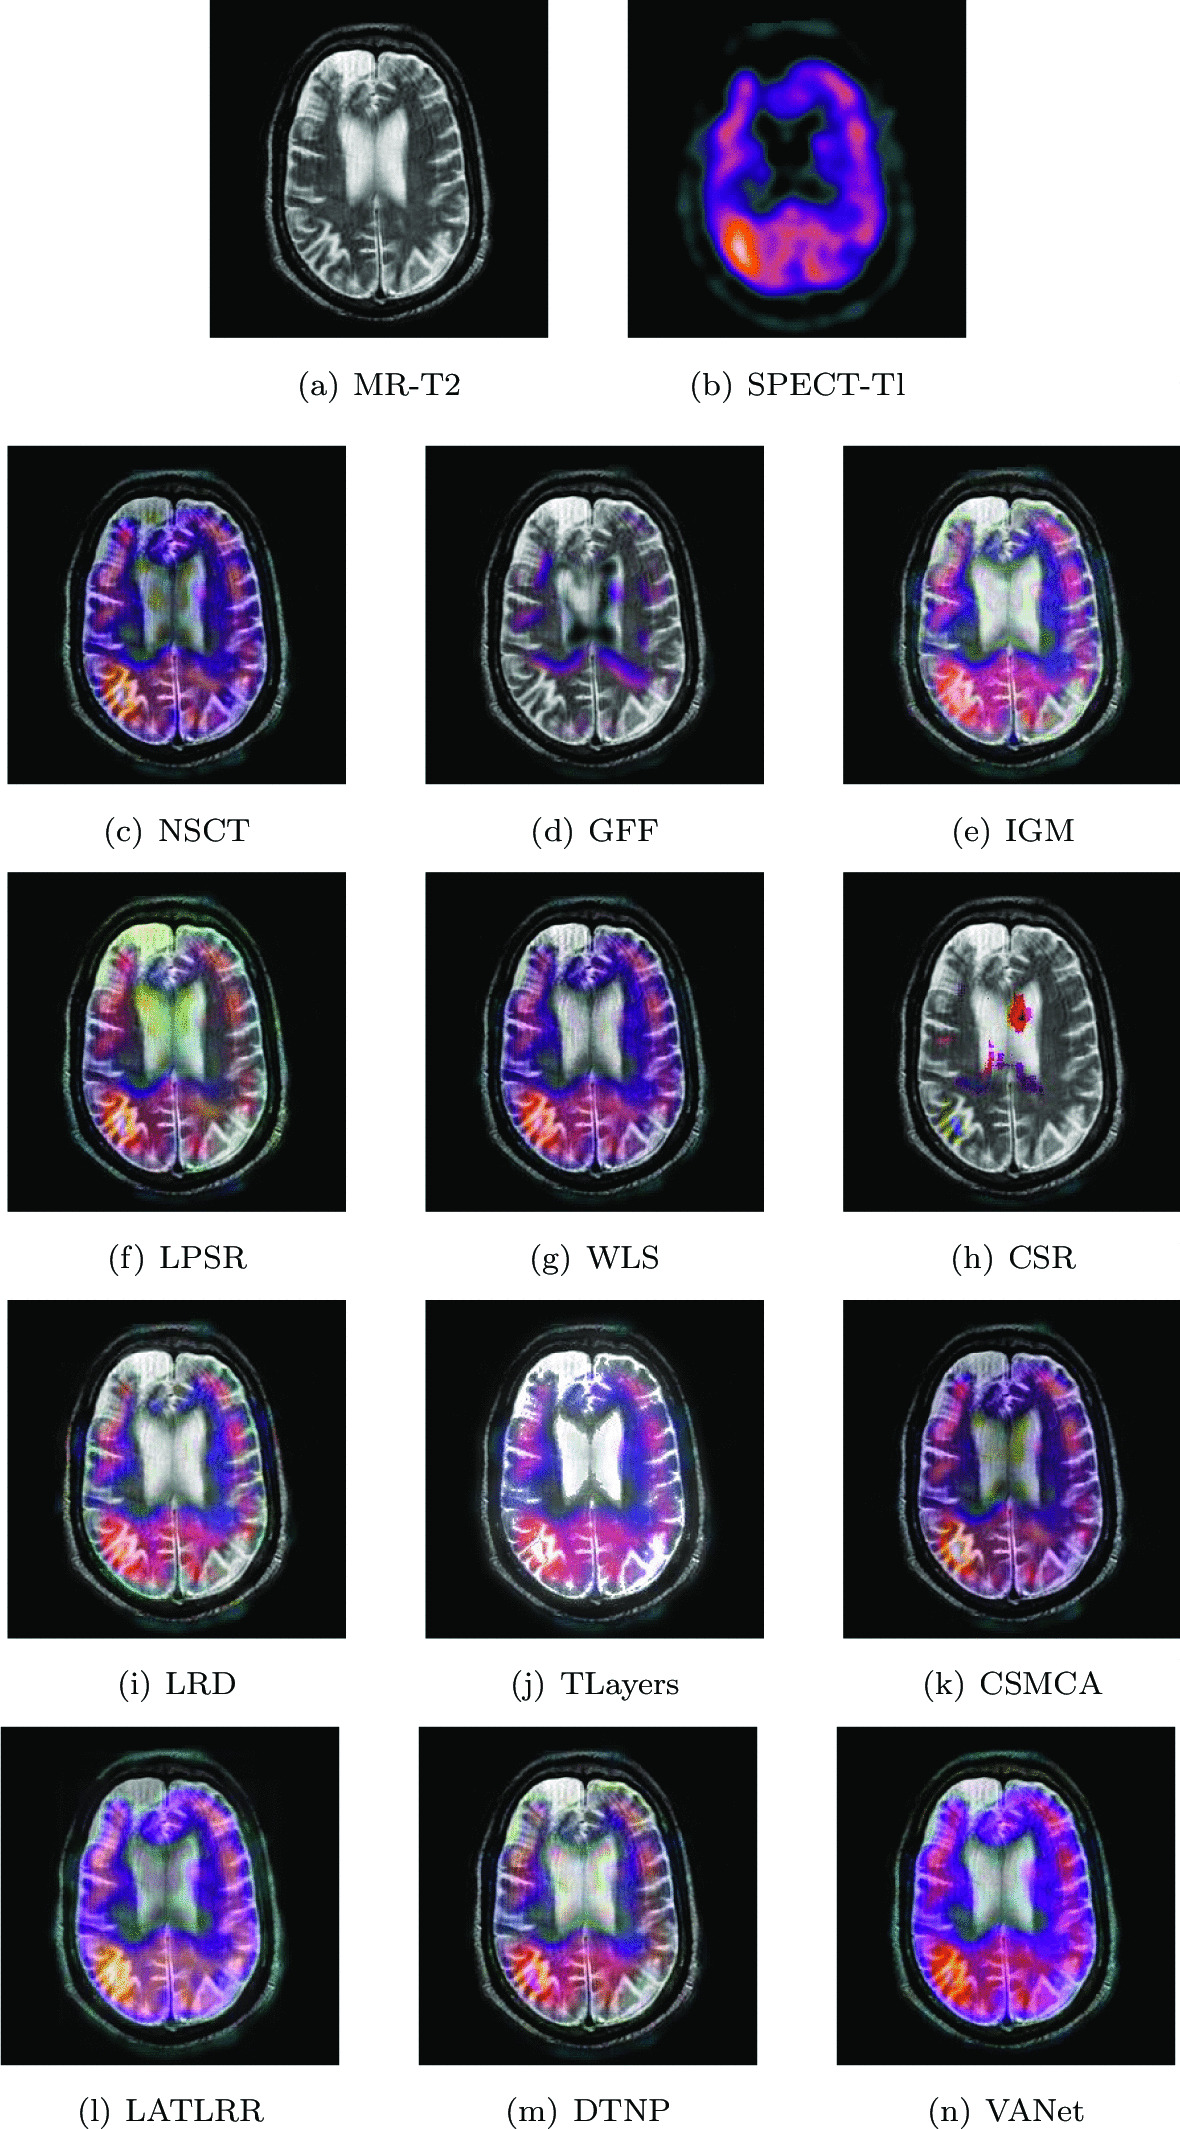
The second set of fused MRI-SPECT images from 9 methods on subacute stroke
